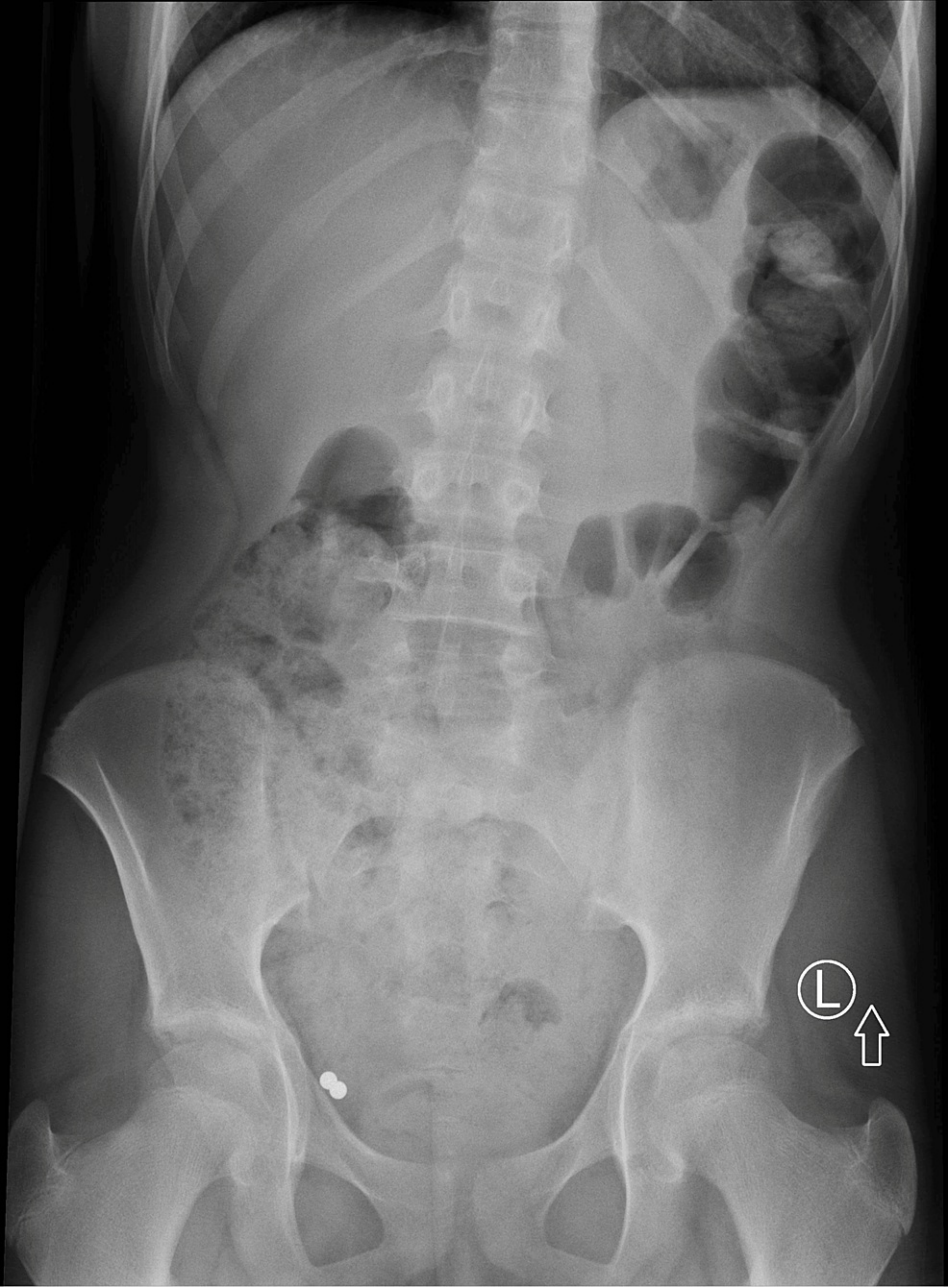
Abdominal X-ray showing two magnetic balls in the right lower quadrant.
